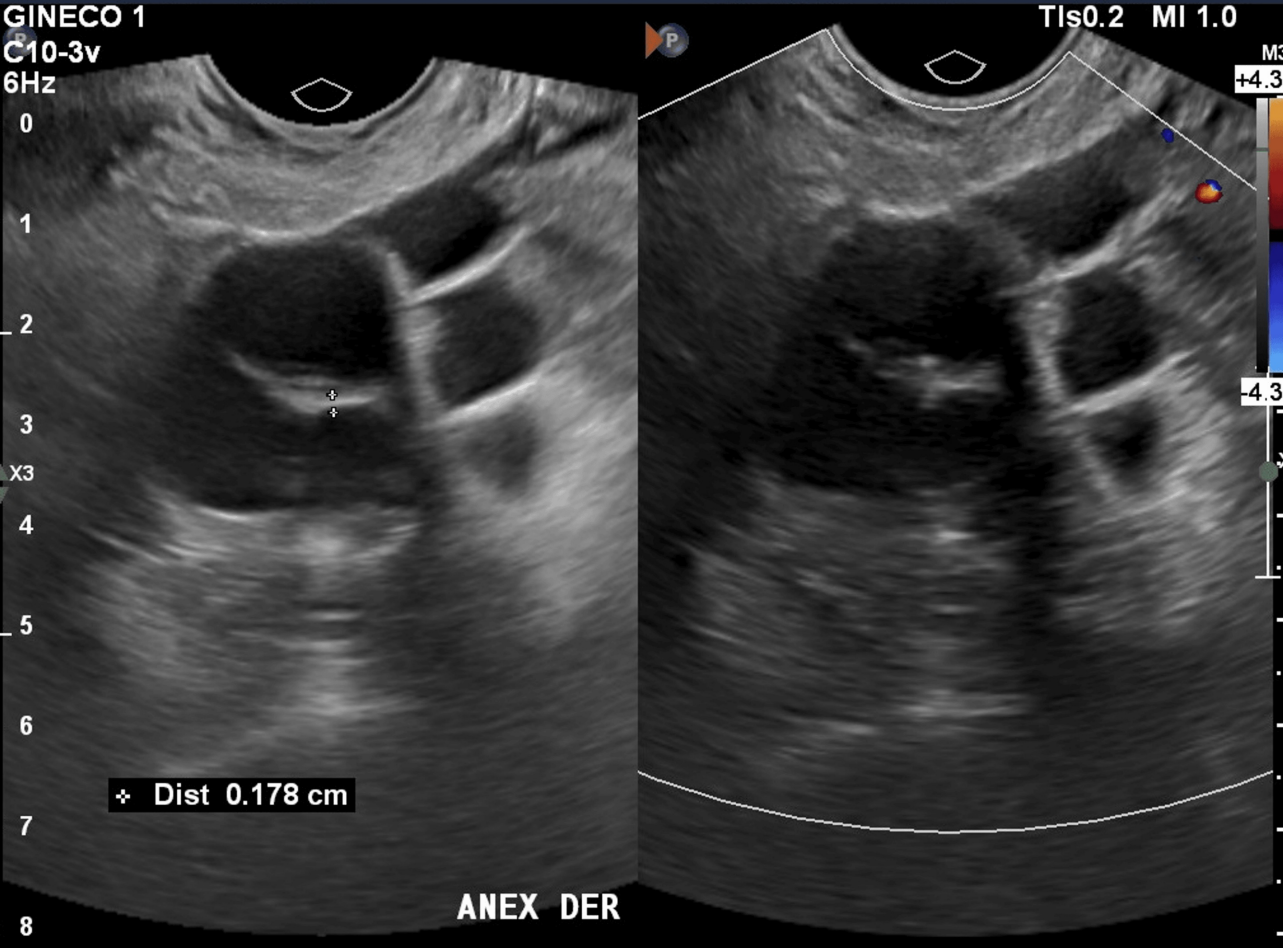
Transvaginal pelvic ultrasound showing a right ovarian mass with well-defined borders and heterogeneous echotexture.
